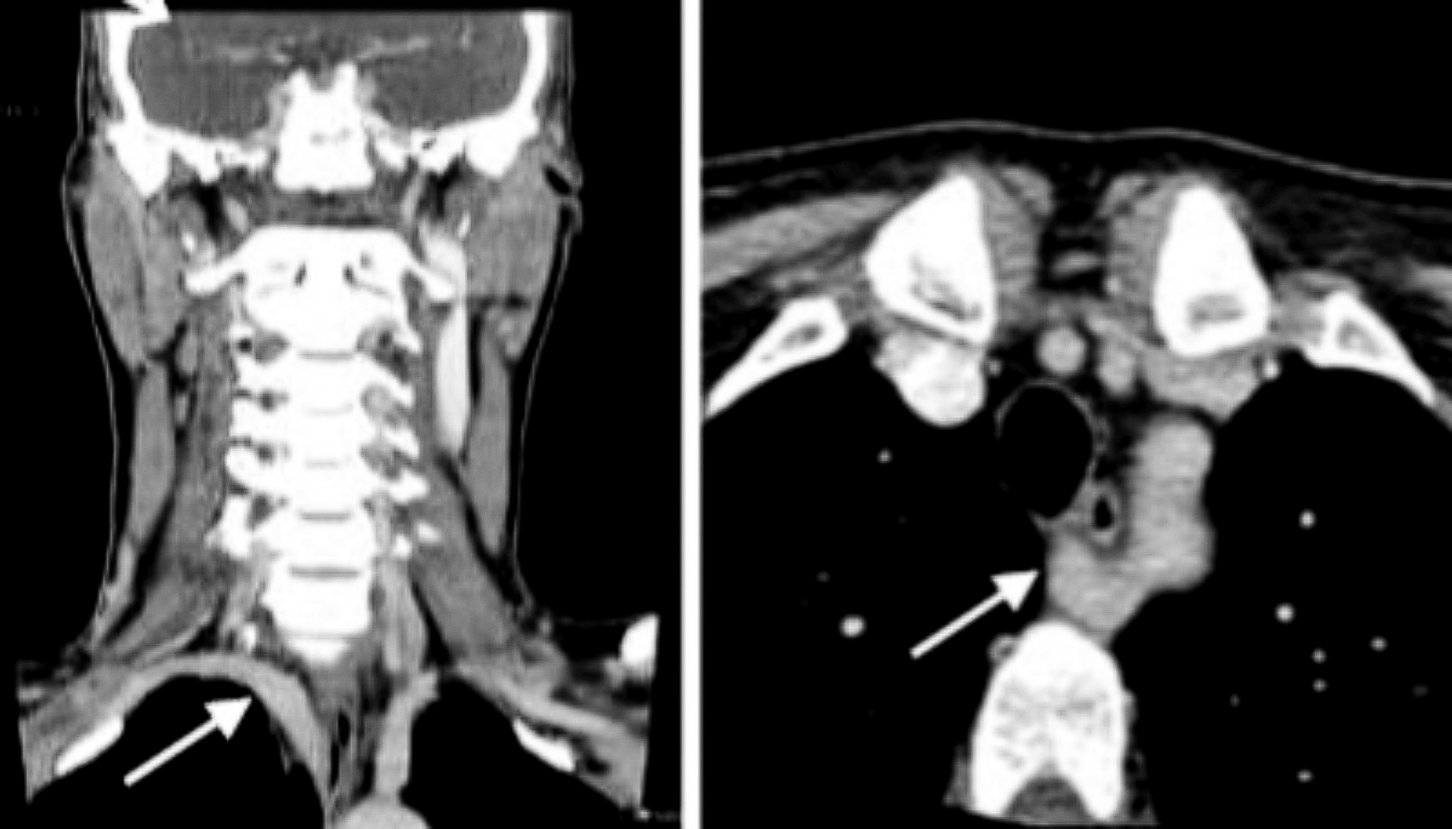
CT of arteria lusoria In the absence of the brachiocephalic trunk, the right subclavian artery emerges directly from the left side of the aortic arch and runs posteriorly to the trachea and esophagus (as indicated by the arrows).
